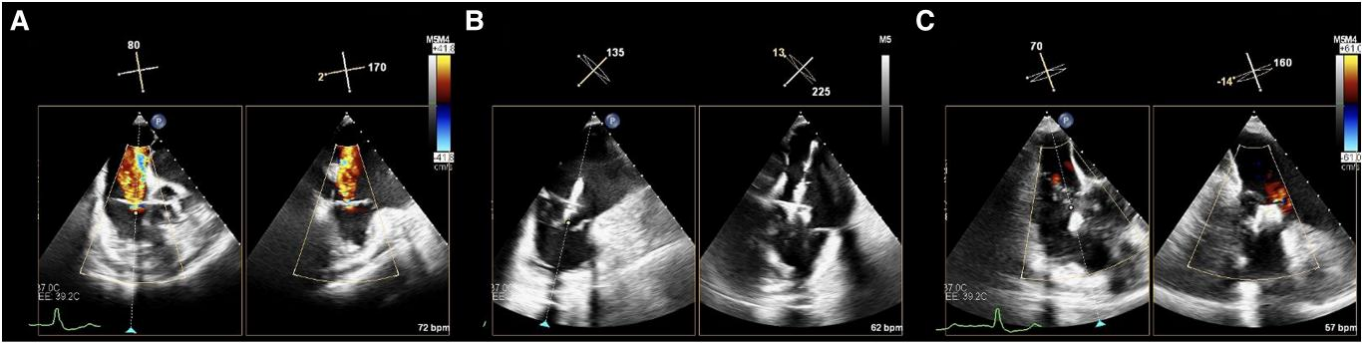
Periprocedural *x*-plane mode transoesophageal echocardiography. (*A*) Tricuspid regurgitation before the procedure, (*B*) during the procedure, and (*C*) after the procedure.
